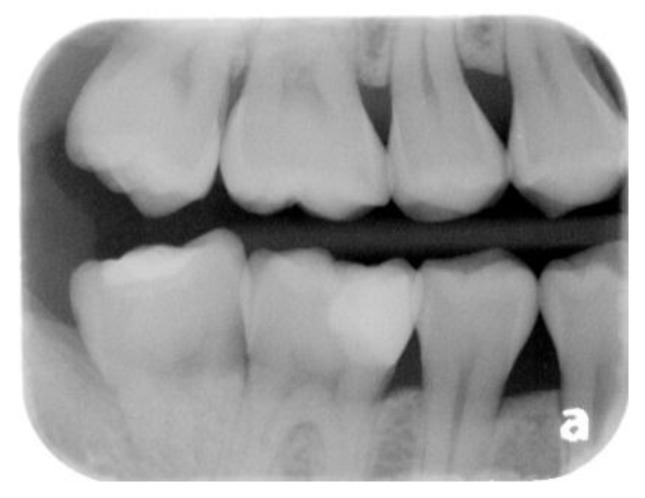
Post-operation bite-wing radiograph shows no obvious overhang or remaining excess resin cement.
